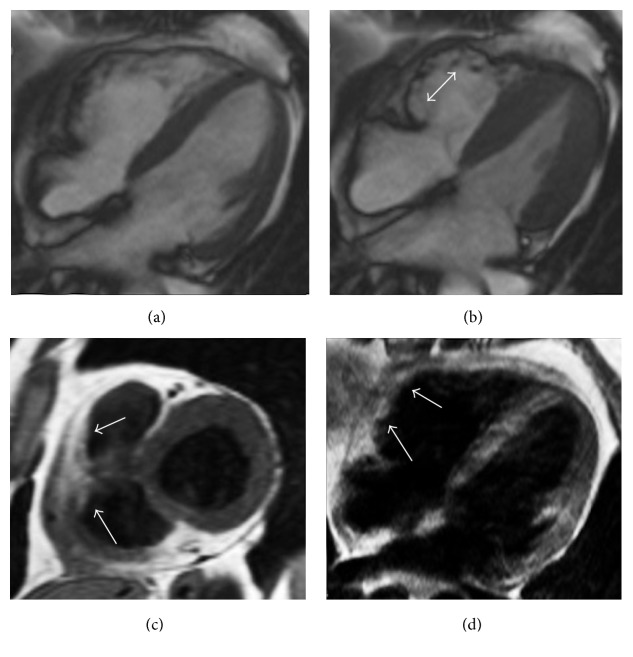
Arrhythmogenic right ventricular cardiomyopathy in a 27-year-old man presenting with palpitations. Cine-SSFP four-chamber images, respectively, in diastole (a) and systole (b) demonstrate an aneurysmatic aspect with dyskinesia of the right ventricular free wall that appears corrugated ((b) double-headed arrow). T1-weighted black blood sequences in short axis (c) and four-chamber (d) views demonstrate adipose infiltration phenomena in the right ventricular free wall (arrows).
